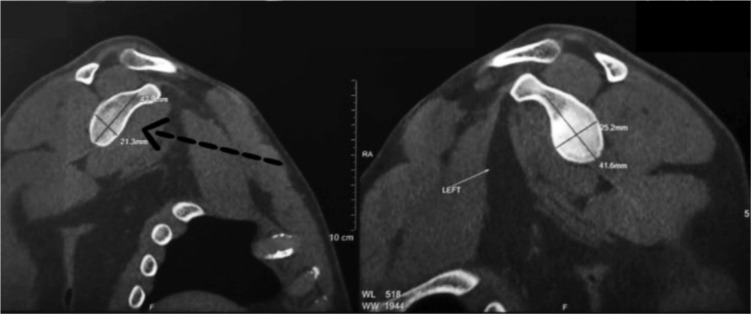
Pre-operative CT showing the glenoid attrition on right side compared to left.
